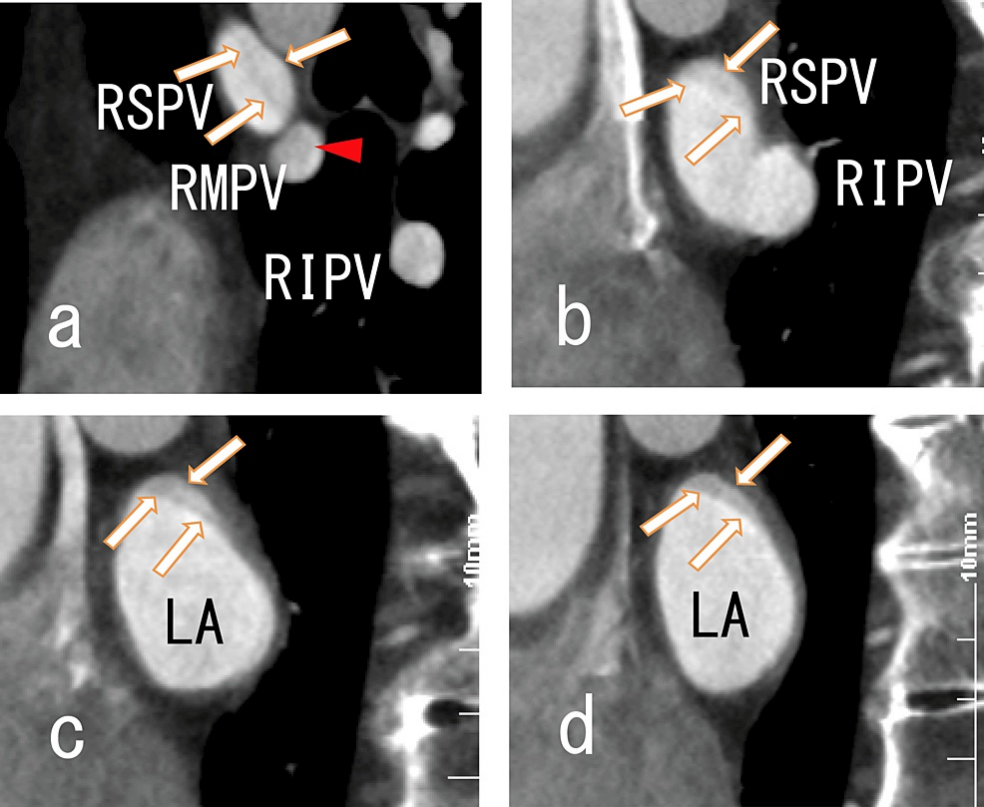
Sagittal images from an 80-MDCT scan showing thrombi in the RSPV and LA Sagittal images from an 80-MDCT scan showing the RSPV, RMPV, RIPV and right side of the LA. There were some images of thrombi in the RSPV, RMPV, RIPV and LA. No LADs were identified. (a) There were rather dark areas in the RSPV (arrows), suggesting that there were thrombi in the RSPV. There were rather dark areas in the RMPV (arrowhead), suggesting that there were thrombi in the RMPV. There were dim dark areas in the RIPV. (b) After the RSPV’s connection to the RMPV, the RSPV then linked to the RIPV. There were rather dark areas in the posterosuperior region of the RSPV (arrows), suggesting that there were thrombi in the RSPV. (c) and (d) There were rather dark areas in the posterosuperior region of the LA (arrows), suggesting that there were thrombi in the LA. The thrombi were adhered to the posterosuperior wall of the LA. 80-MCDT, 80-slice multidetector computed tomography; LA, left atrium; RIPV, right inferior pulmonary vein; RMPV, right middle pulmonary vein; RSPV, right superior pulmonary vein; LADs, left atrium diverticula
